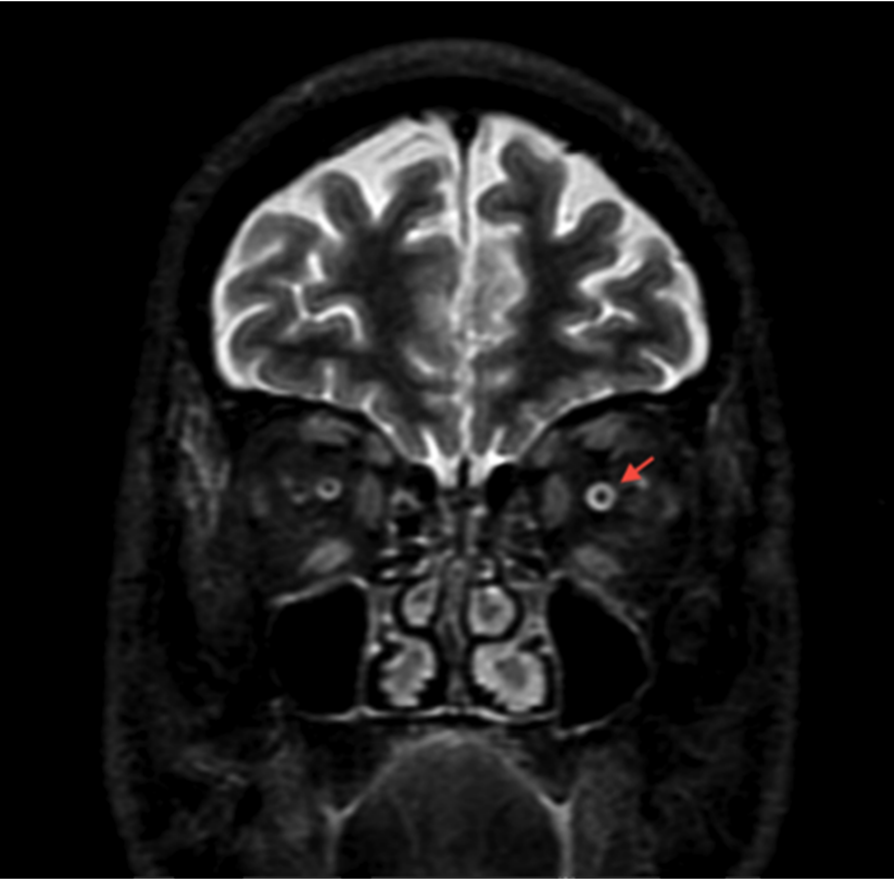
Coronal short tau inversion recovery magnetic resonance imaging scan. Faint hyperintensity of the left optic nerve can be seen in T2 (red arrow).
